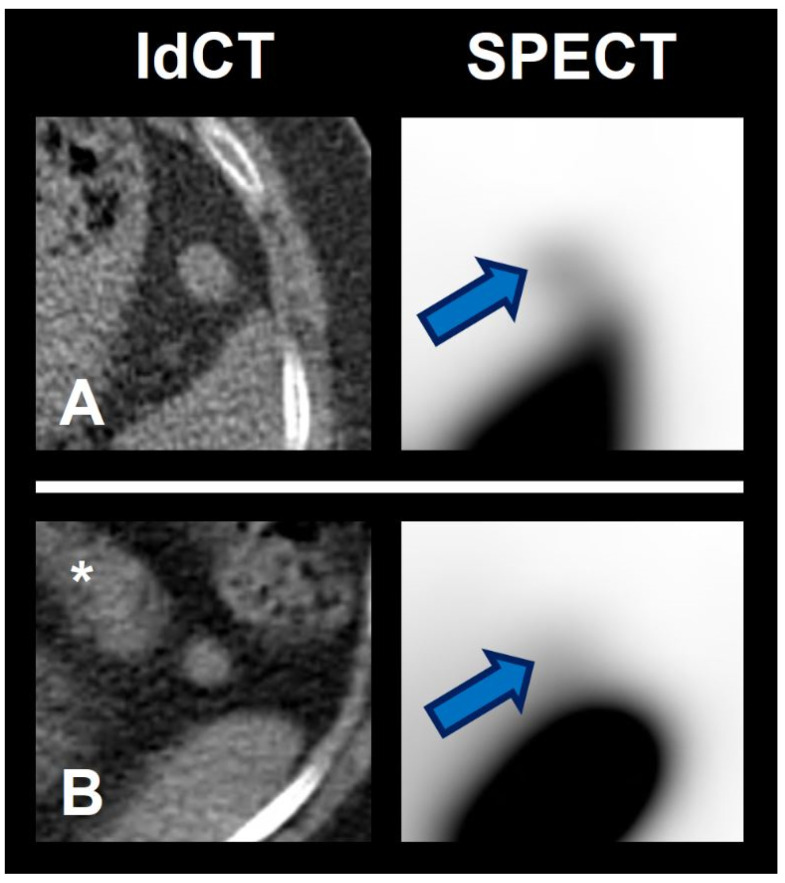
Example of two challenging cases in spleen scintigraphy reading. (Left) low dose CT. (Right) ^99m^Tc-labelled denatured red blood cells SPECT. (A) = Rated as spleen tissue using the quantitative cutoff. Clinical follow up showed no evidence of a progression of the perisplenic lesion. (B) = Not rated as spleen tissue using the quantitative cutoff. Endosonographic biopsy was performed and confirmed the rating. Arrows = lesional uptake. Asterisk = pancreas.
